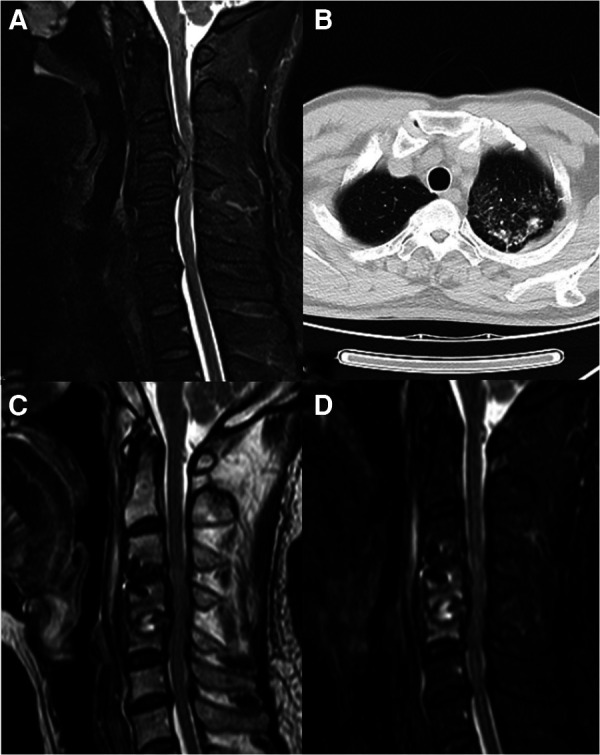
(A) Preoperative T2. (B) Preoperative chest CT. (C) Two months postoperative T2. (D) Two months postoperative fat reduction image.
